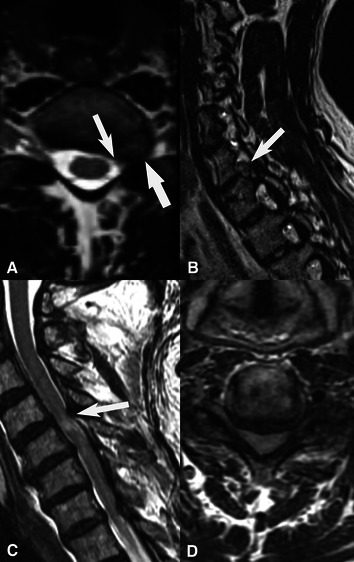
MRI of two different patients that had a history of ulnar nerve compression with a diagnosis resulting in surgical treatment of the nerve compression disorder at the elbow, with and without another nerve compression disorder. (A,B) T2W axial and sagittal images showing left sided foraminal disc herniation C7-Th1 (thick arrow) with compression of the C8-nerve root (thin arrow, A). (C,D) T2W sagittal and axial images of another patient with severe central stenosis with total obliteration of CSF spaces around the spinal cord (D) and signal changes in the spinal cord at level C4-C5 (thin arrow C).
